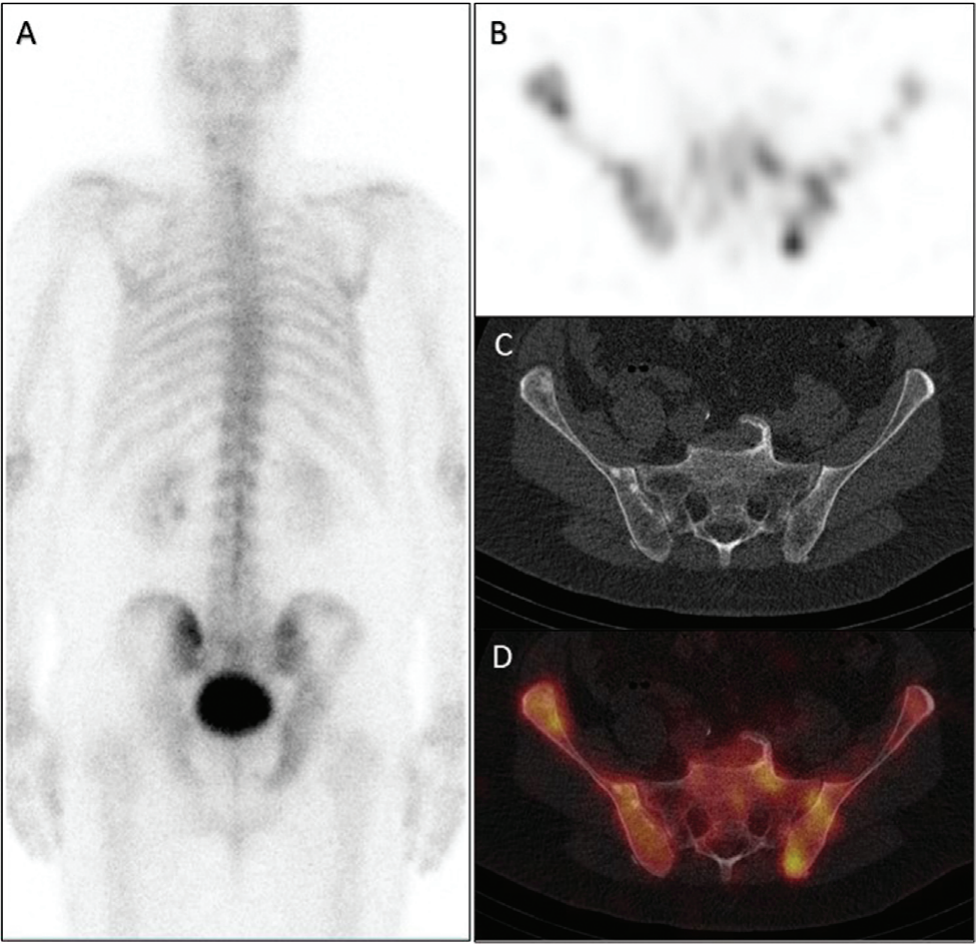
Whole body bone scan and single-photon emission computed tomography/computed tomography.
